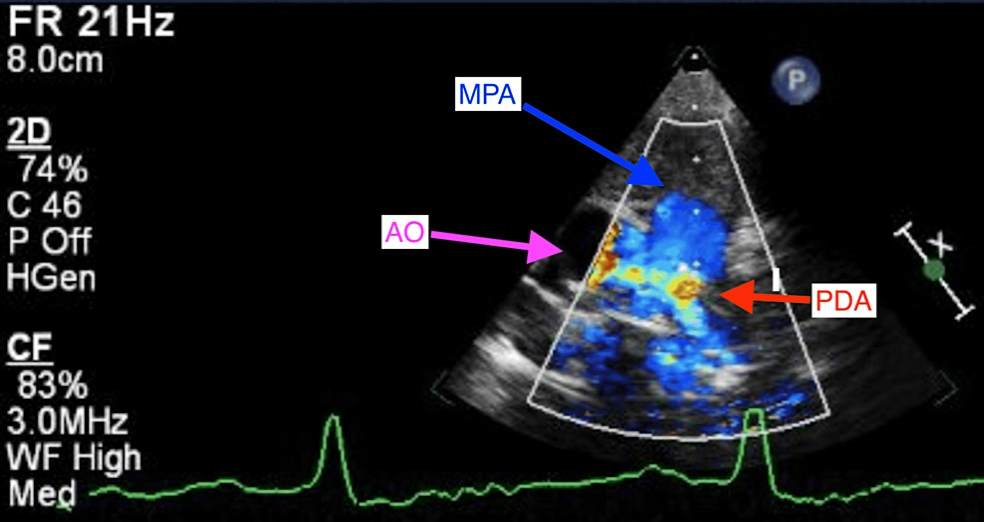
Echocardiography parasternal short-axis view at the aortic valve level with color flow doppler showing turbulent flow of PDA (yellow mosaic signals) with left to right shunting. AO: aorta; MPA:main pulmonary artery; PDA: patent ductus arteriosus
